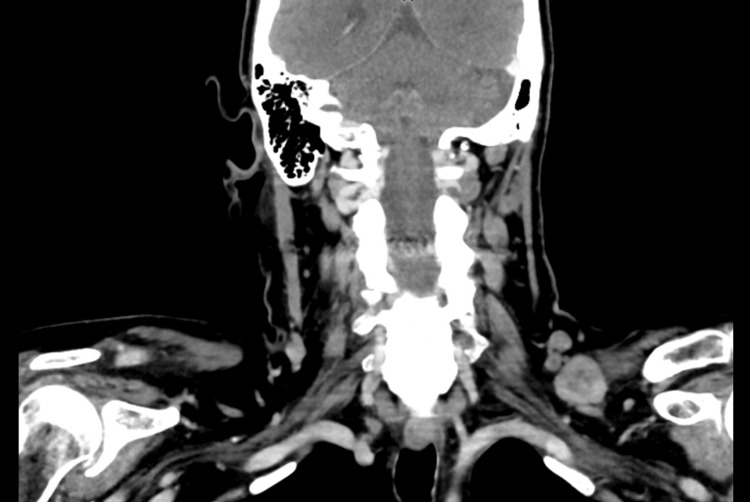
CT soft tissue neck with IV contrast, showing an enlarged left supraclavicular lymph node within a red square, measuring approximately 3.2 x 2.3 cm. CT: computed tomography
